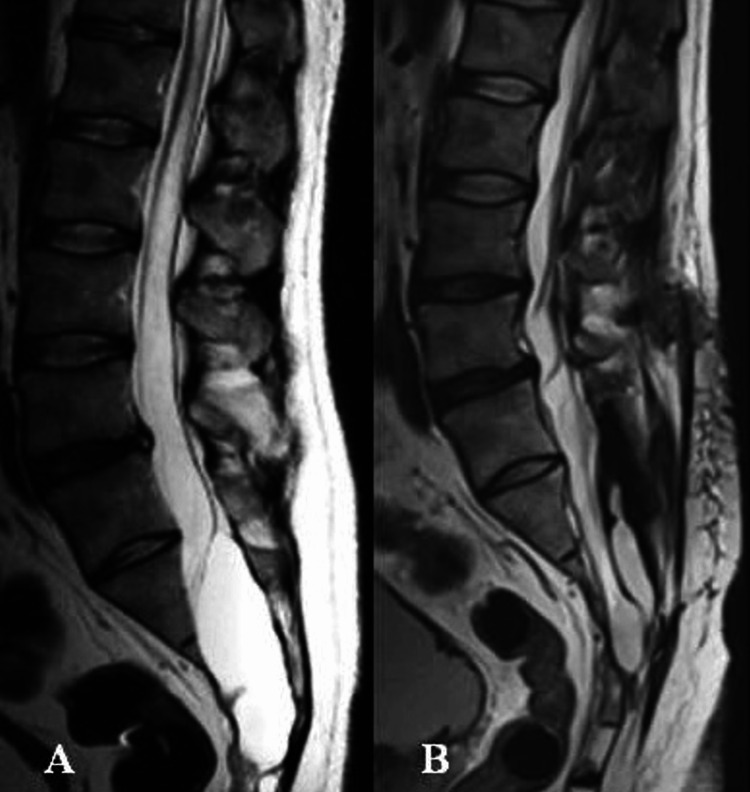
Comparisons of MRI of the patient with sacral intradural arachnoid cyst at S1-S5 level. (A) Preoperative; (B) Six years after surgery.
